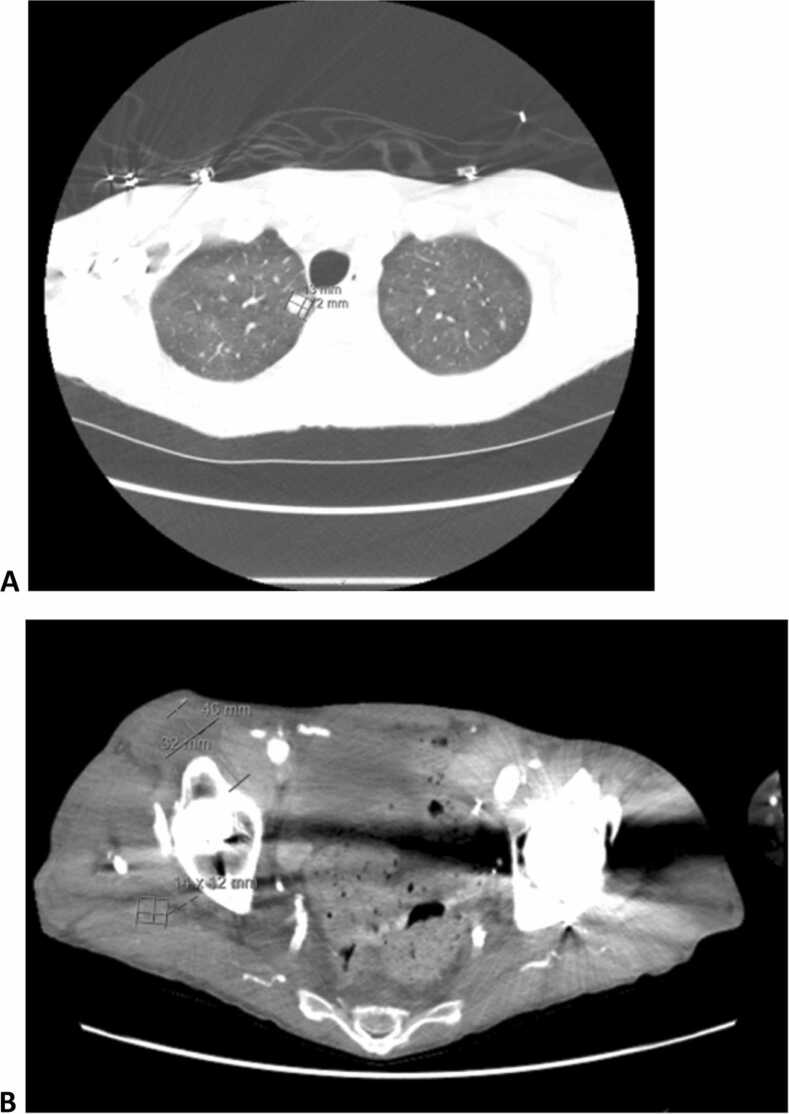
(A) CT Chest revealing right cavitary lung nodule. (B) CT Pelvis showing thick-walled fluid collections anterior and posterior to the right hip joint.
